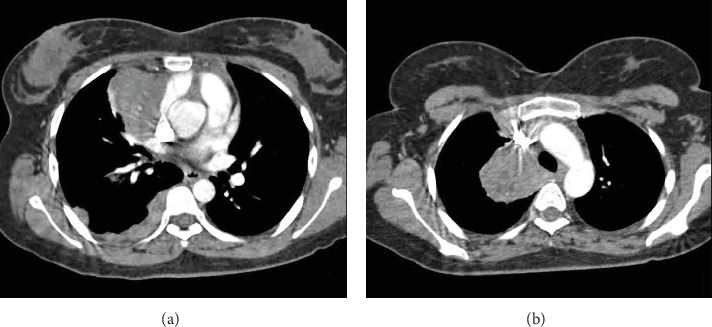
(a) Computed tomography image of the chest depicting a large right upper paratracheal mass. (b) Computed tomography image of the chest depicting pleural and pericardial.
